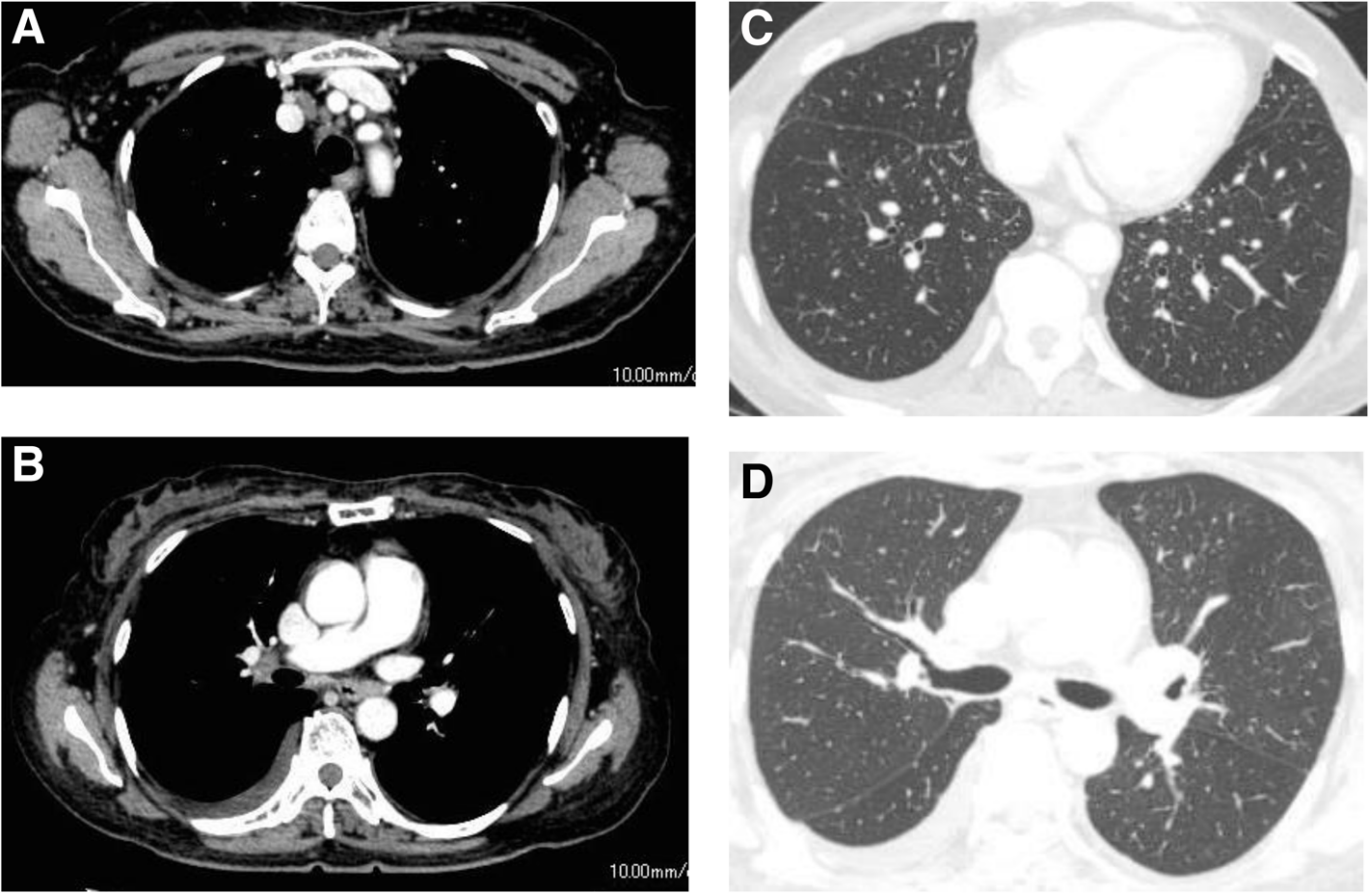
Chest computed tomography findings after 5 weeks of corticosteroid therapy. a-d The multiple mediastinal and bilateral hilar lymphadenopathy, bilateral pleural effusion, small nodules in the lung and the interlobar pleura, and small subcutaneous nodules have regressed
